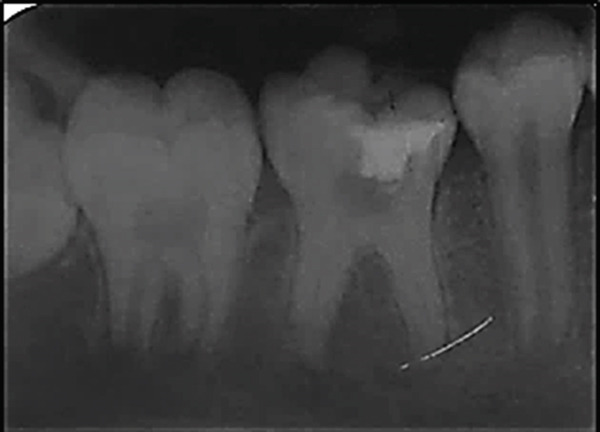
Preoperative radiograph of 46.
